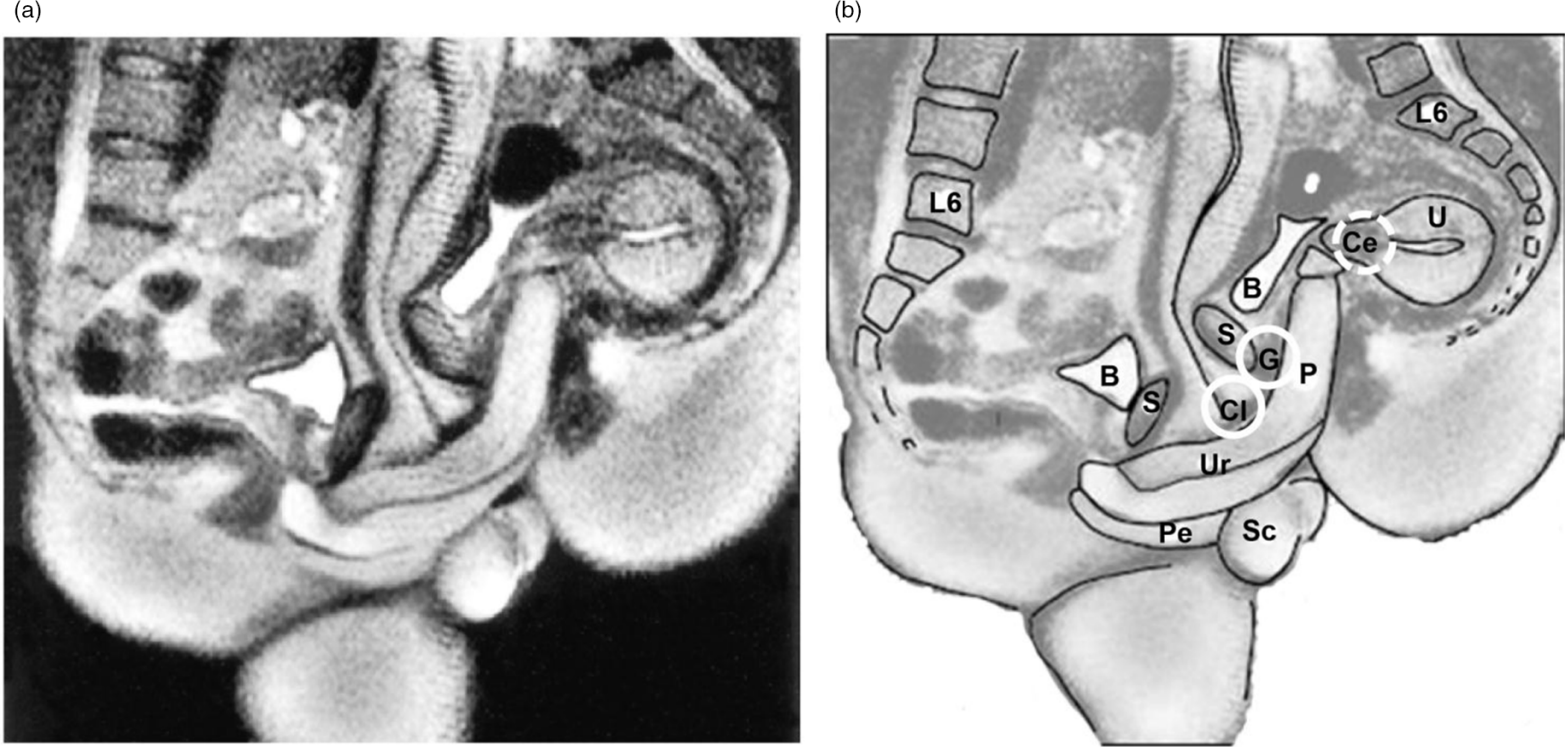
Magnetic resonance image (MRI) of a penis inside a vagina. Participant couple was in the ‘missionary position’. Note the ‘boomerang’ curvature of the penis to meet the shape of the vagina. Left: MRI image. Right: image with approximate anatomical locations of external clitoris, internal clitoris or ‘G-spot,’ and cervix, as in Fig. 4. Images adapted from Schultz, van Andel, Sabelis, and Mooyaart (1999) and reprinted with permission. P, penis; Cl, clitoris; G, G-spot; Ce, cervix; Ur, urethra; Pe, perineum; U, uterus; S, symphysis; B, bladder; Sc, scrotum.
